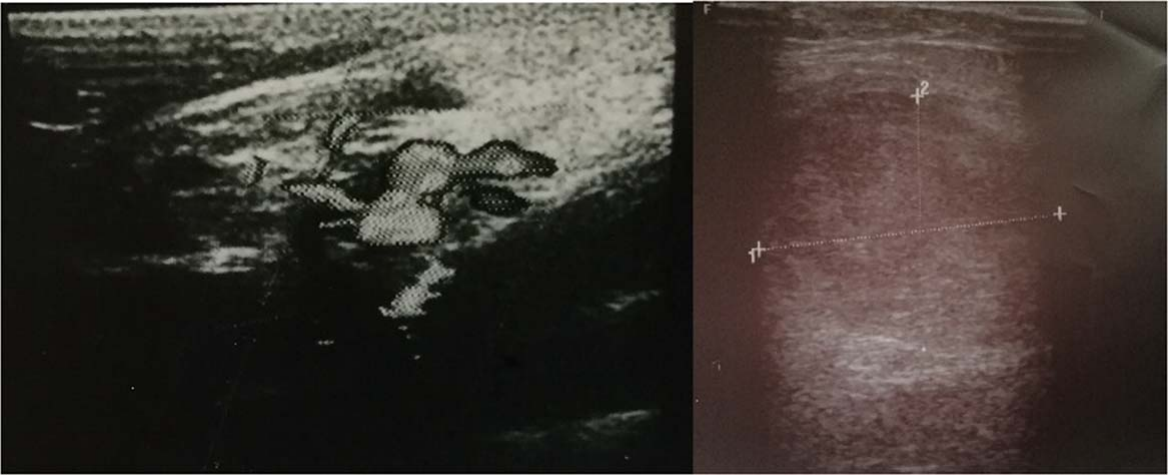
Ultrasound revealed an accumulation of hematoma in the right calf.
